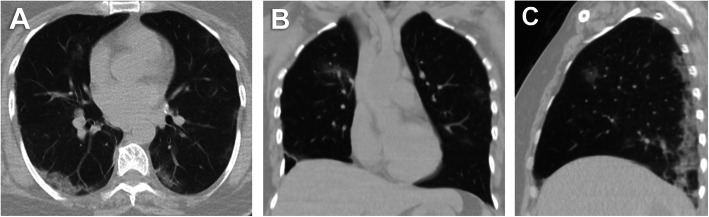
A 50-year-old female patient. A axial, B coronal, and C sagittal chest CT images show bilateral peripheral ground-glass opacities and consolidation with mild pulmonary involvement (5–25%) corresponds to current chest CT score 8 and simple chest CT score 2 (mild affection). The patient survived
